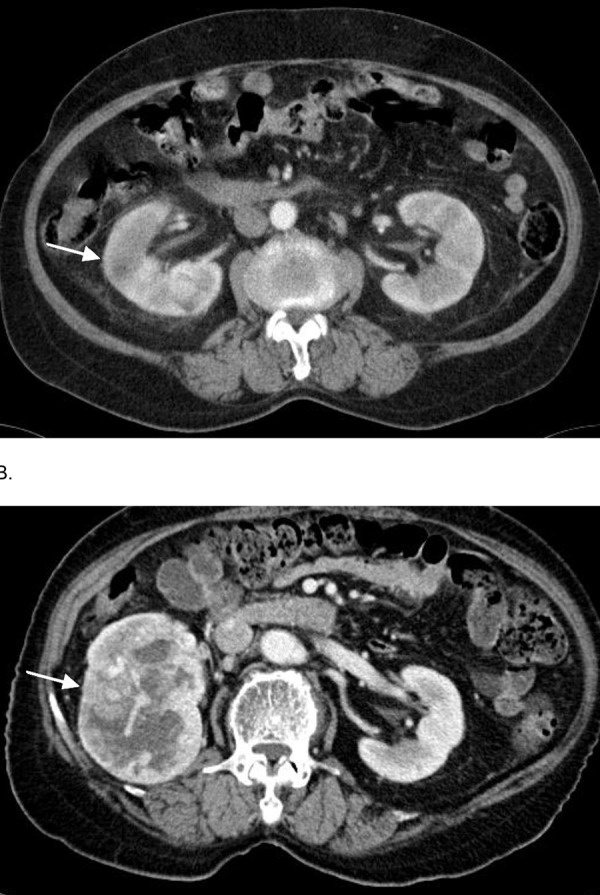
Figure 1. A: Computed tomography (CT) of the abdomen and pelvis demonstrated some hypoenhancing lesions with perinephric strandings in both kidneys and a suggestive small abscess in the right kidney (arrow), which was compatible with acute pyelonephritis. B: 5 years after the treatment of acute pyelonephritis, CT of abdomen and pelvis indicated an exophytic 6 × 8 cm sized mass with necrosis in the mid and lower portion of the right kidney (arrow).
